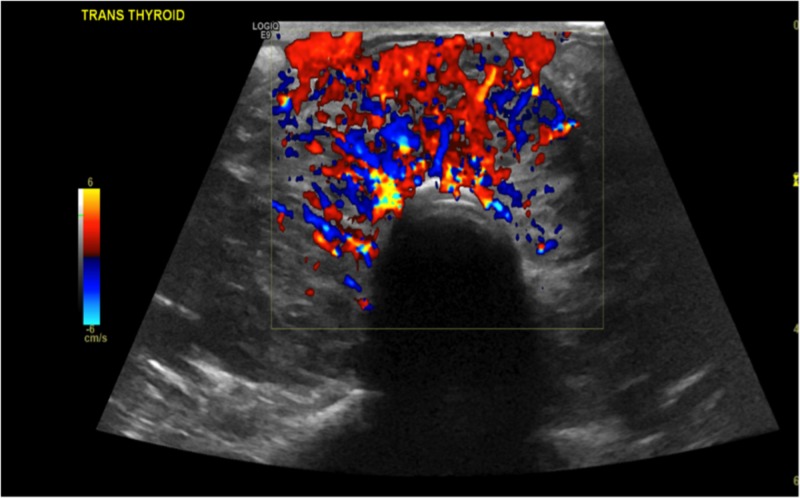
Doppler showing vascularity in the isthmus of thyroid
